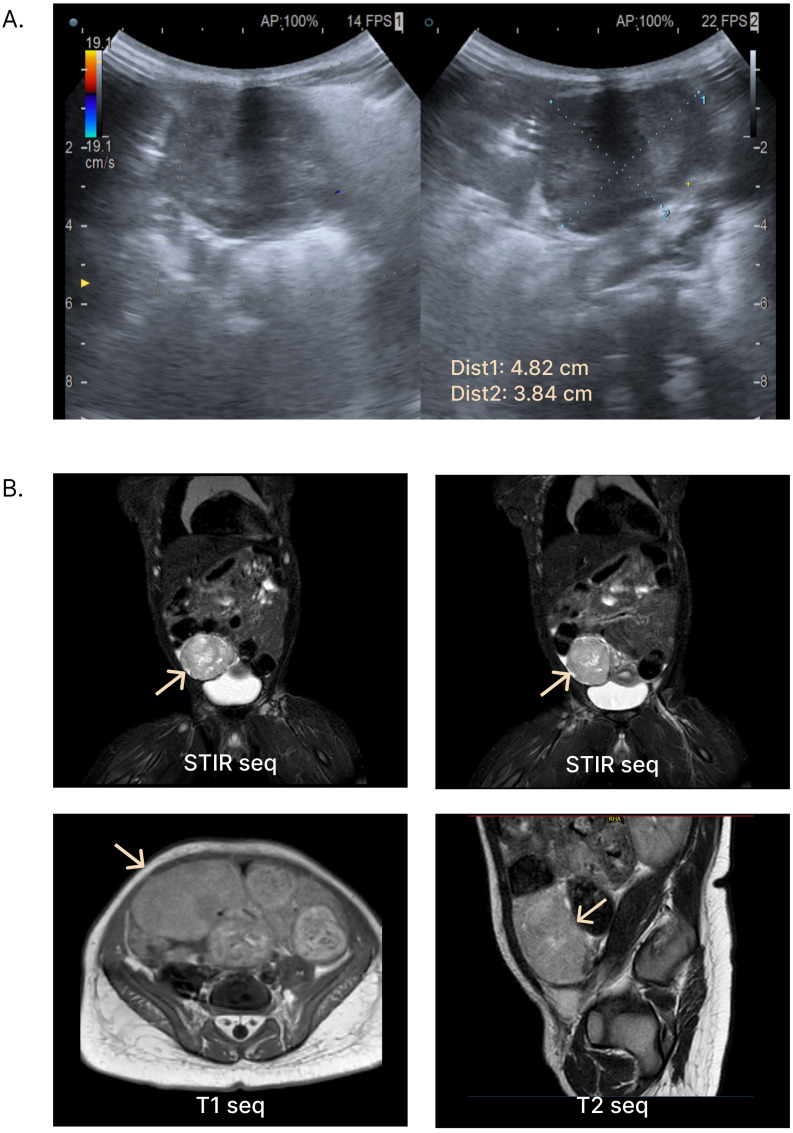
Radiologic diagnostic testing. (A) Ultrasound (US) images obtained at diagnosis, revealing a pelvic mass in the right flank region. (B) Contrast-enhanced abdominal and pelvic MRI images showing a right pelvic mass originating from the left adnexa, with features suggestive of neoplastic etiology. *Dist*, *distance; Seq*, *sequence*.
